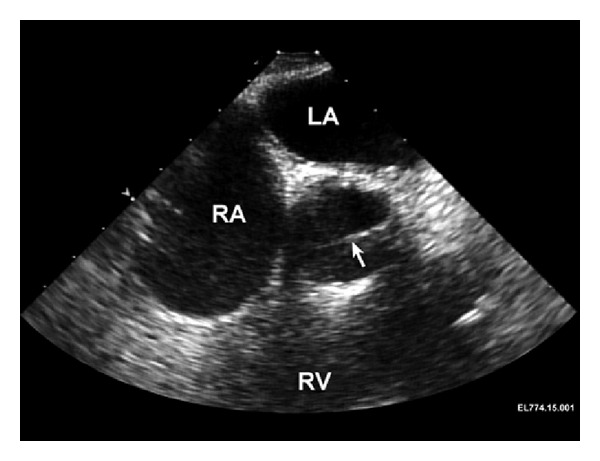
Intraoperative transesophageal echocardiographic view prior to replacement of the aortic valve prosthesis. This midesophageal aortic valve short axis view during systole demonstrates the normal open position of one of the mechanical aortic valve leaflets (arrow). The left atrium (LA), right atrium (RA), and right ventricle (RV) are also identified.
